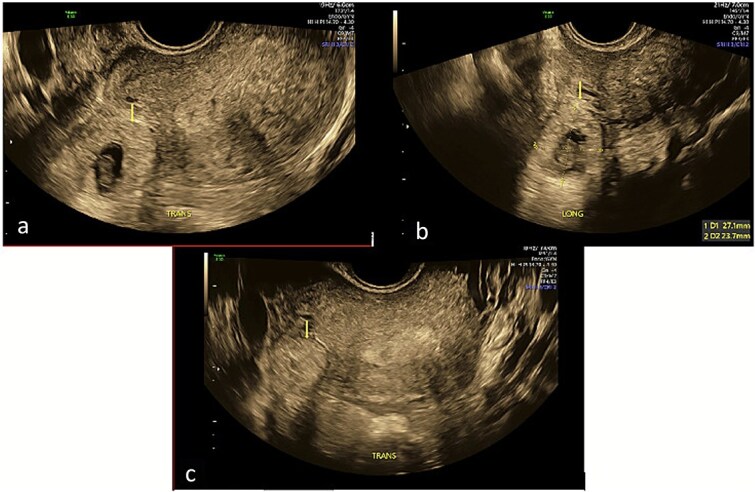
Transverse and longitudinal views of the uterus on trans-vaginal ultrasound demonstrating a right interstitial ectopic pregnancy with gestational sac and foetal pole. Image A demonstrates a decidual reaction around the gestational sac in a transverse plane through the uterus. Image B measures the gestational sac in the longitudinal plane. Image C demonstrates that the pregnancy does not communicate with the endometrial cavity in a transverse plane through the uterine fundus.
